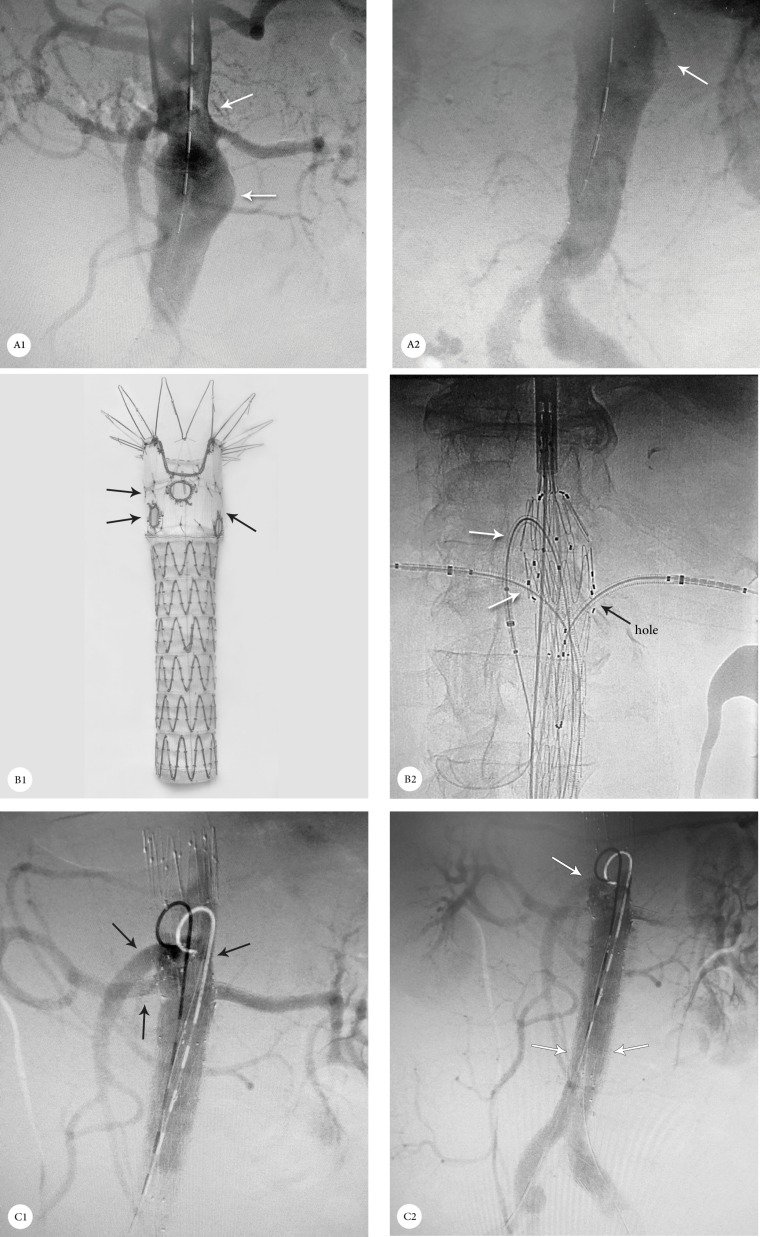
A1 & A2: Computed tomography angiography, showing a thoracoabdominal aneurysm (arrows); B1: Location of the holes (arrows) in the custom-made 3-hole stent graft; B2: Fluoroscopy in the anterior posterior view, showing the successful cannulation from the 3 holes of the stent graft; C1 & C2: Digital subtraction angiography following fenestrated endovascular aortic aneurysm repair with the exclusion of the aneurysm (white arrows) and perfusion of the celiac artery, superior mesenteric artery, and both renal arteries (black arrows).
